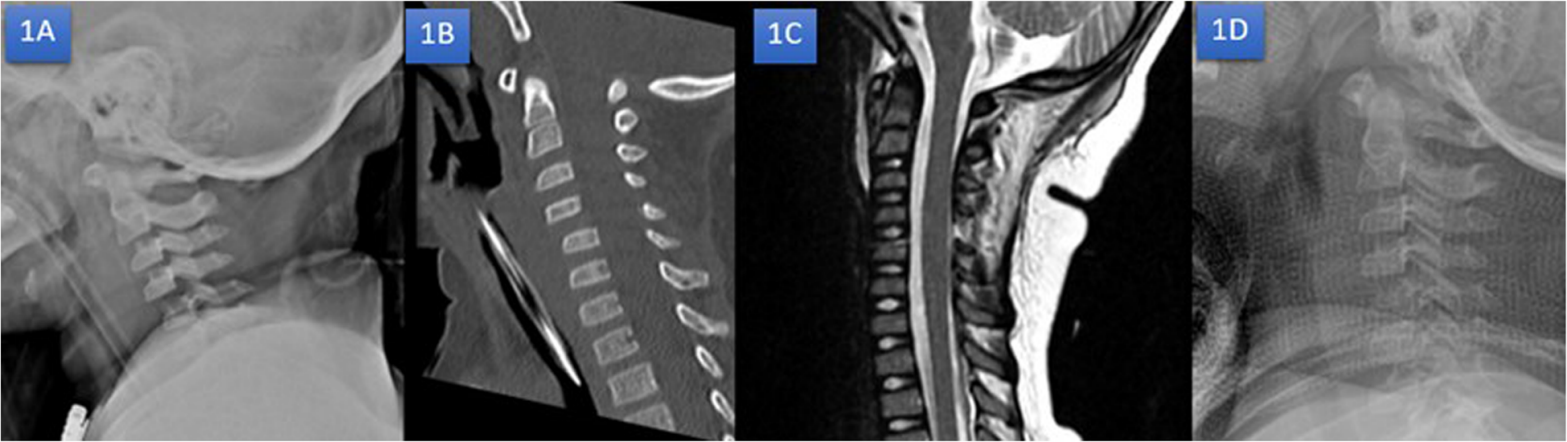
Radiographs images; Lateral radiograph x-ray (1A), sagittal radiograph of CT (1B) and sagittal radiograph of T2 weighted MRI (1C) showed antrolesthesis of C4 on C5 with 25% translation without spinal cord injury. Repeated lateral radiograph x-ray after closed reduction maneuver with minerva casting (1D)
